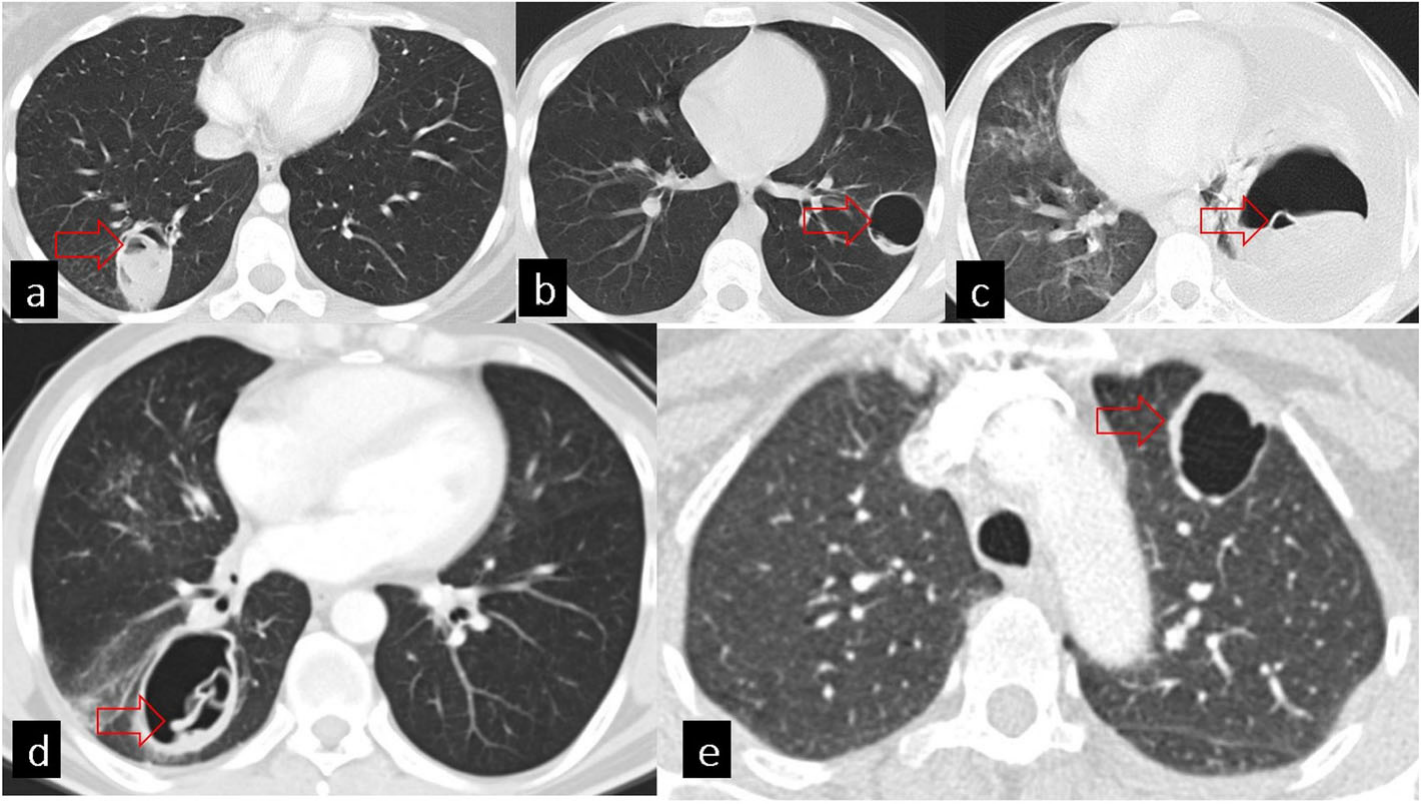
Complete rupture signs. Axial CT images of different patients on the lung window (a–e). a Cumbo sign (red arrow). b Mass within cavity sign (red arrow). c Waterlily sign (red arrow). d Whirl sign (red arrow) e. Empty cyst sign (red arrow)
